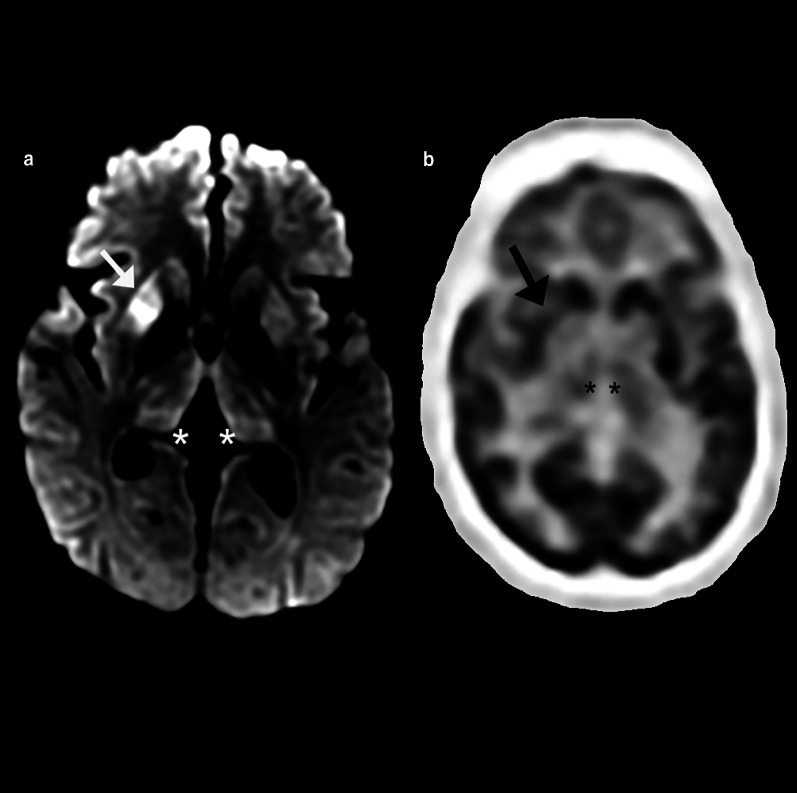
A 64-year-old female with three months of progressive functional decline, failure to thrive, and weakness. Diffusion-weighted imaging (a) demonstrates hyperintensity in the right caudate head and lentiform nucleus (arrow) and the bilateral thalami with an L-shaped or “hockey stick” configuration (asterisks). Fluorodeoxyglucose PET/MRI (b) demonstrates corresponding asymmetric hypometabolism in the right lentiform nucleus (arrow) and bilateral thalami (asterisks). Cerebrospinal fluid 14-3-3 protein was positive and pathological examination of the brain was consistent with CJD
